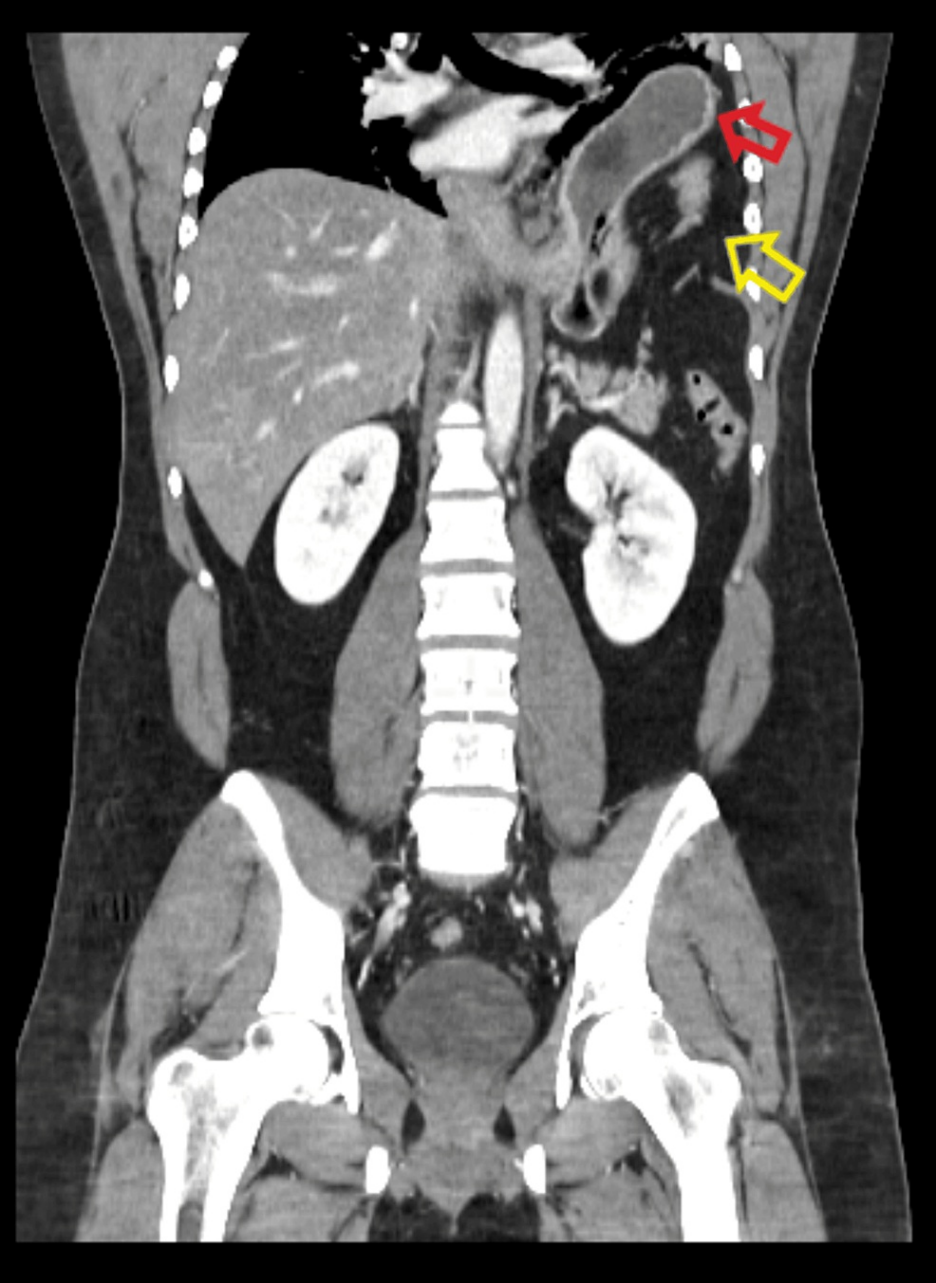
Computed tomography scan done at the initial presentation (coronal view) showing left-sided diaphragmatic hernia, with the stomach (red arrow) and the omentum (yellow arrow) in the left hemithorax
